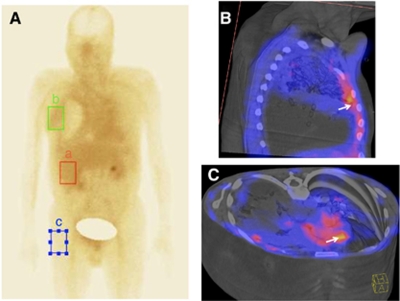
(A) Whole-body scan acquired 1 h after ^99m^Tc-LD injection. The image shows the ROIs as described in the text by the (a) Liver, (b) Tumoural tissue, and (c) Soft tissue (background area). Single-photon emission CT images in figures (B) and (C) show ^99m^Tc-LD uptake in tumour tissue (arrows).
